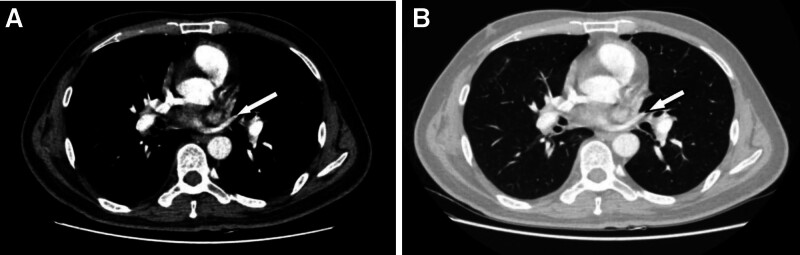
CT showed a special variant of the S4 vein. (A, B) Lung and mediastinum window shows a separate regurgitation of the S4 segment of the left upper lobe of the lung into the left atrium (indicated by the white arrow). CT = computed tomography.
